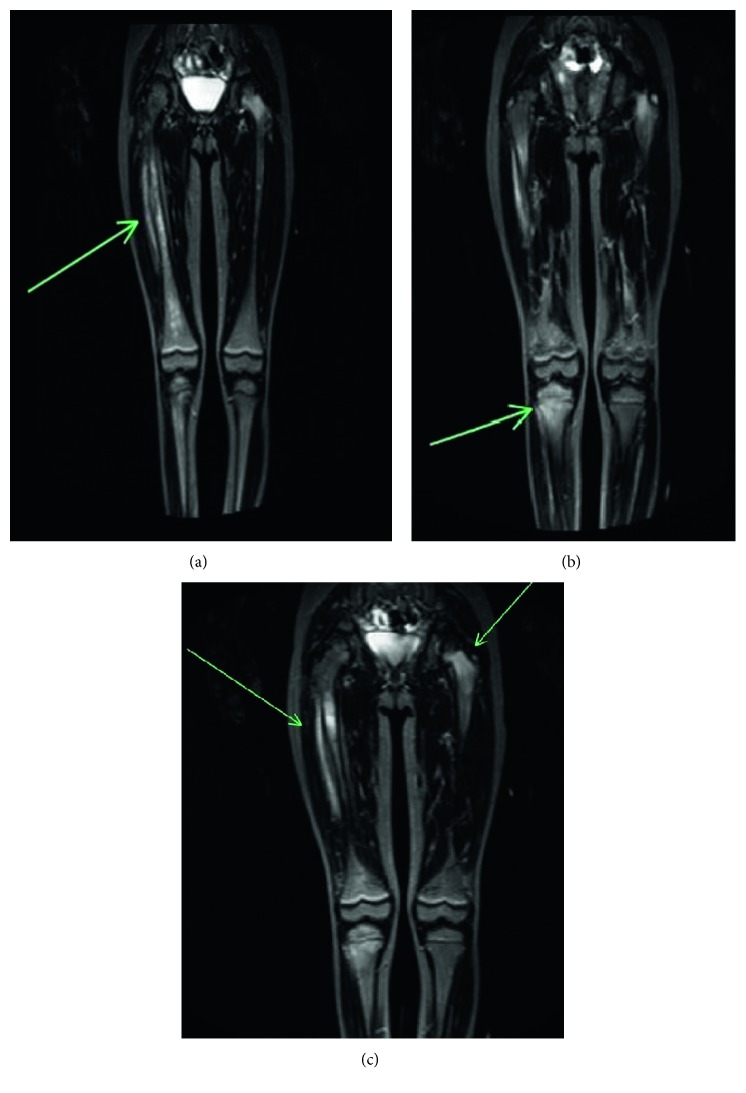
MRI lower extremities showed multifocal abnormal bone marrow signal in the right femur, the left femoral neck, and the proximal epiphysis and metaphysis of the right tibia.
